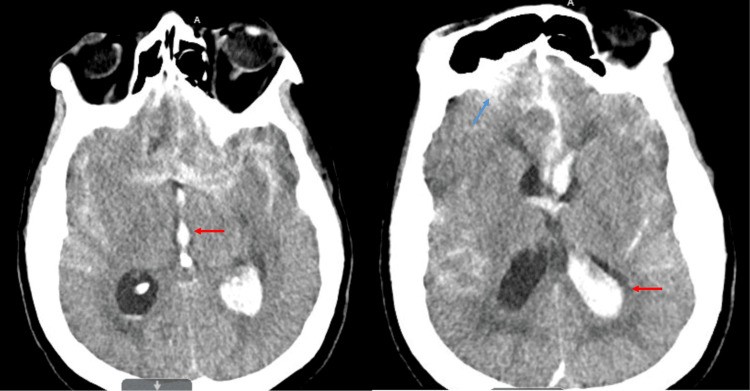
Head CT of patient Head CT of 73-year-old female who was found unresponsive by EMS with a low level of consciousness. There is evidence of extensive subarachnoid hemorrhage in basilar cisterns with extension into the ventricles (red arrows). Also pictured is a 2.4 x 2 x 1.3 cm intraparenchymal hemorrhage in the right frontal lobe (blue arrow).
